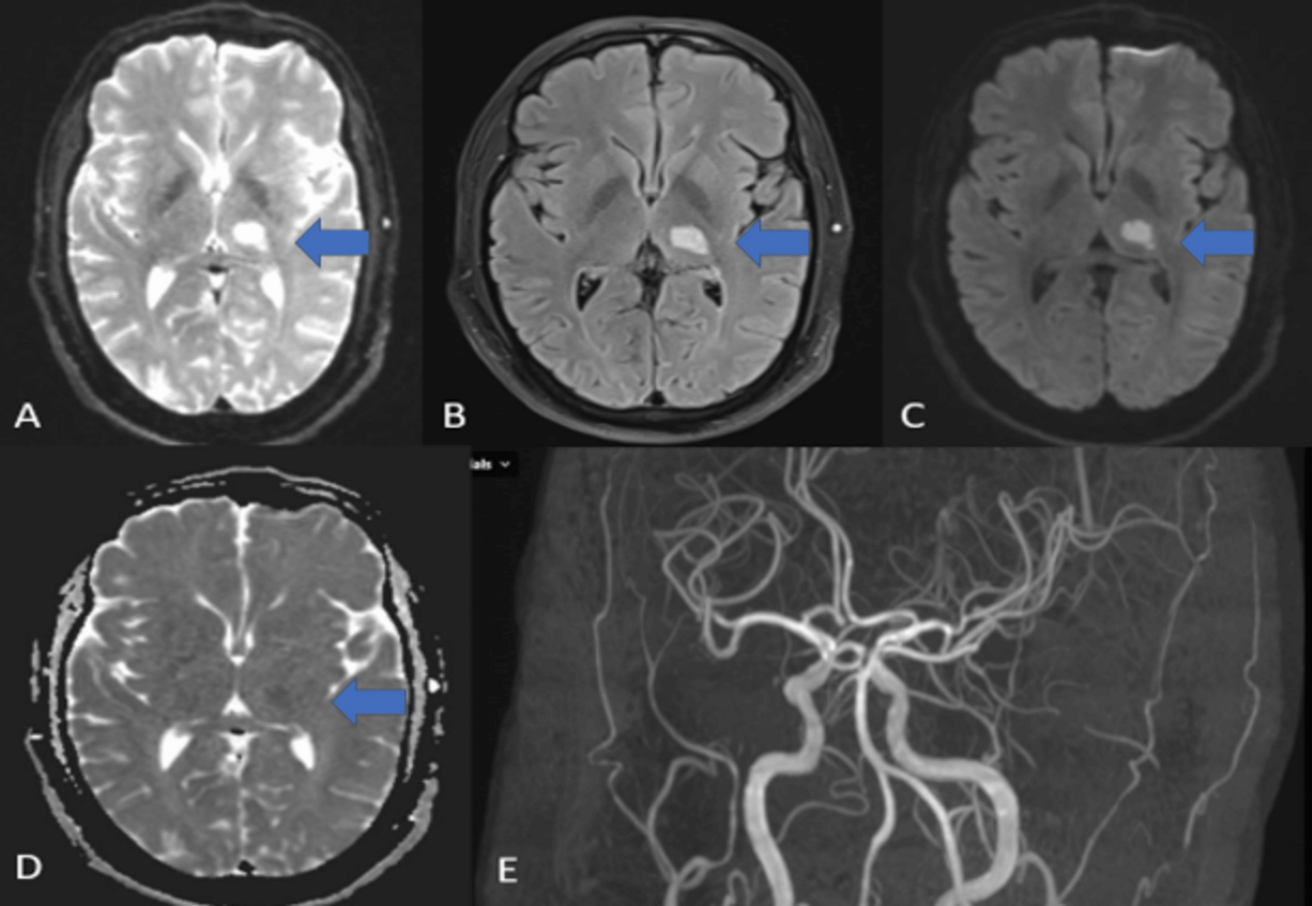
(A) T2 weighted imaging; (B) T2 FLAIR MR imaging shows a hyperintense lesion (blue arrow) in the left thalamus; (C) DWI shows an increased signal in the left thalamus (blue arrow); (D) Reversal on the corresponding ADC map as shown by (blue arrow); (E) 3D TOF MR angiogram revealed normal cerebral vasculature FLAIR: fluid-attenuated inversion recovery; DWI: diffusion-weighted imaging; ADC: apparent diffusion coefficient; TOF: time of flight
